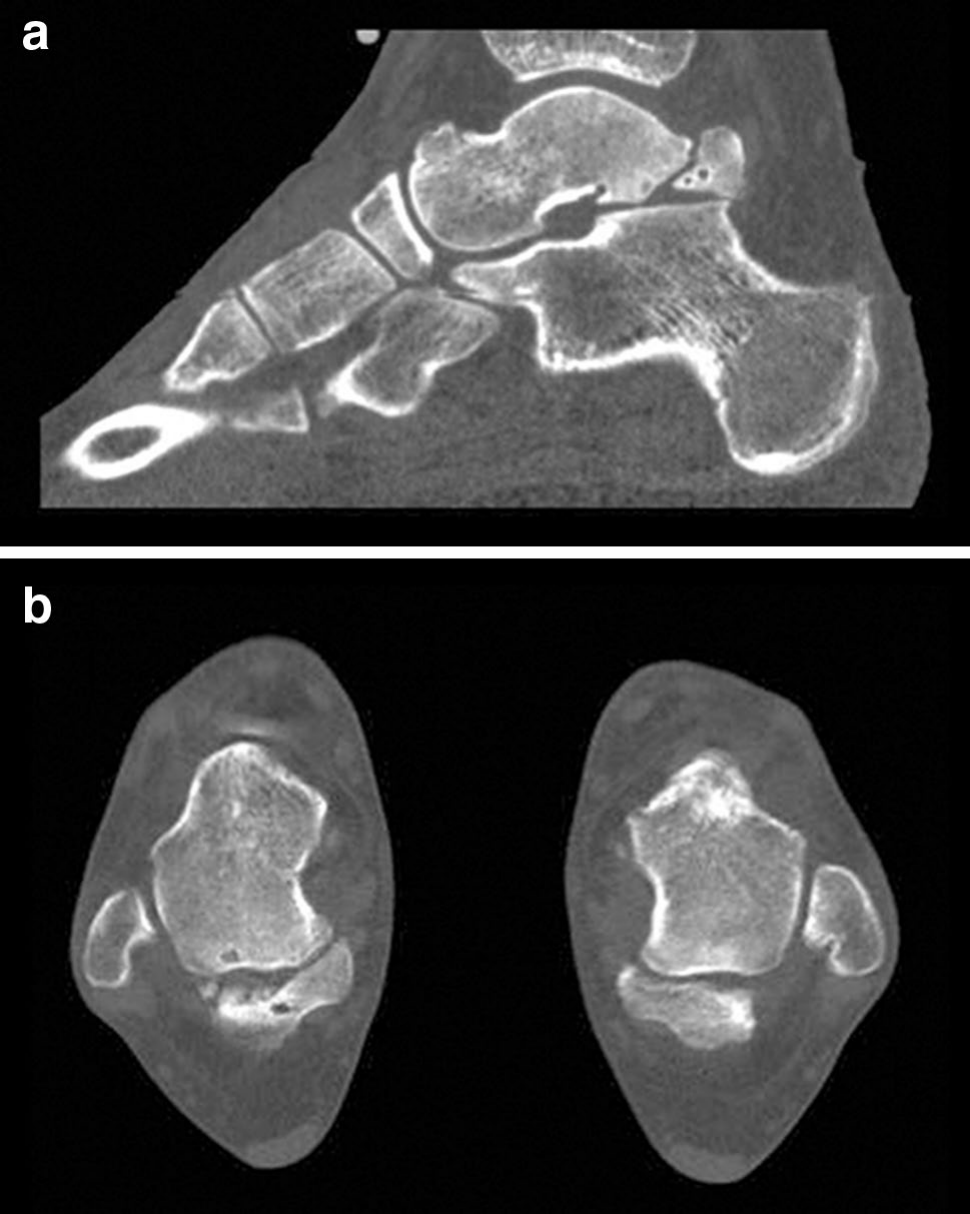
CT scan case 1. a Sagittal view of the left ankle showing a talus bipartitus. b Axial view showing a bilateral talus bipartitus
